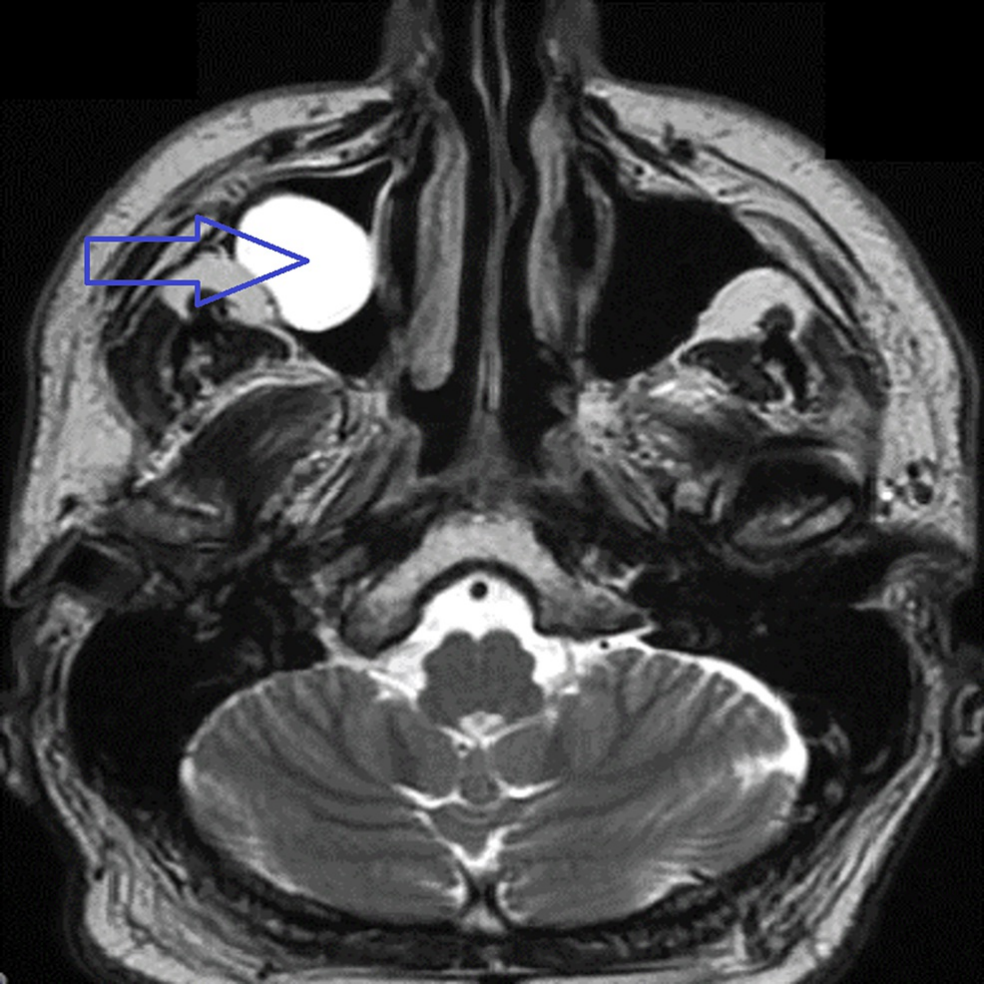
Axial T2-weighted image reveals retention cyst in the right maxillary sinus (arrow).
